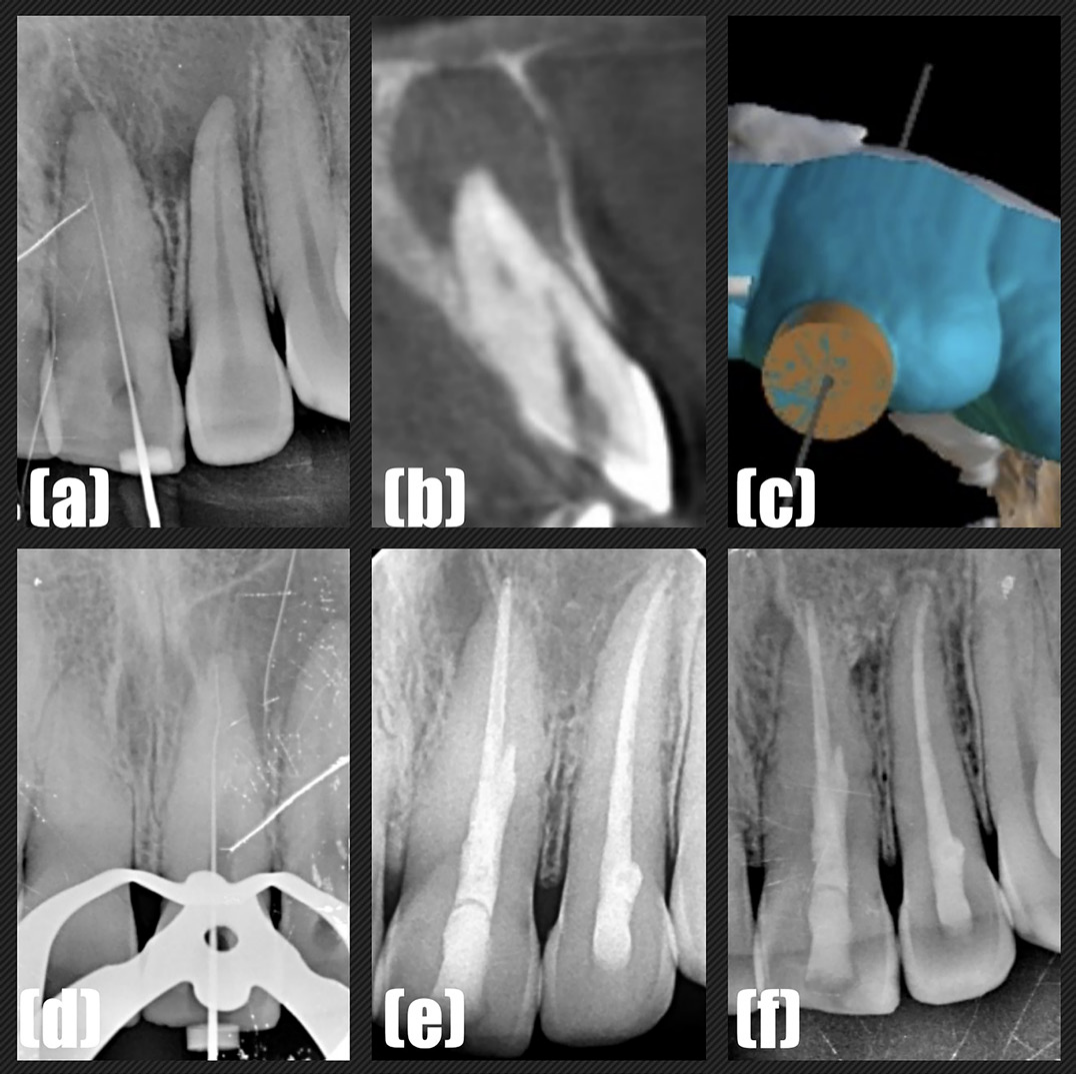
(a) The periapical radiography showing the presence of perforation. (b) CBCT showing the presence of perforation on the labial root wall. (c) The guide designed and 3D printed. (d) The periapical radiography which confirmed the working length. (e) Postoperative periapical radiograph. (f) Follow-up radiograph one year after the completion of the root canal treatment.
